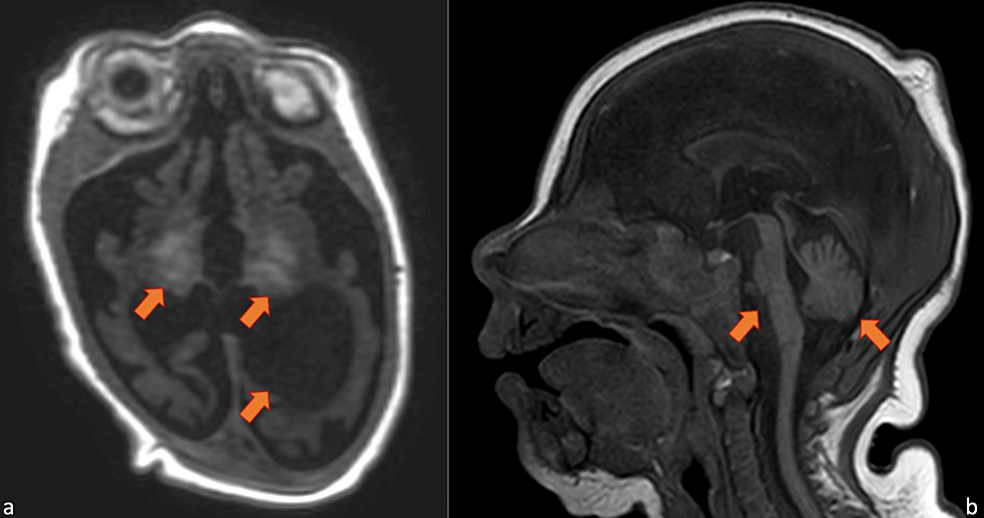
Brain MRI at follow up Brain magnetic resonance imaging on month 6 of life. Hydrocephalus, further cortical cerebral atrophy and focal hyperintensity in the basal ganglia on axial T1-weighted imaging (a); marked cerebellar and midbrain atrophy on sagittal T1-weighted (b).
